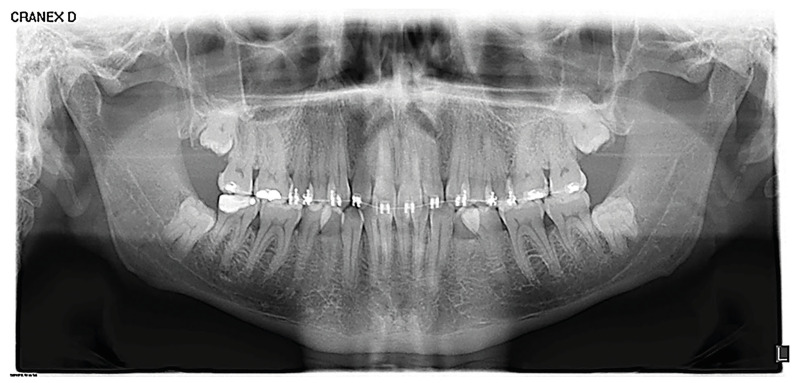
Panoramic radiograph number 25 used in the survey for evaluation of MTM 38.
